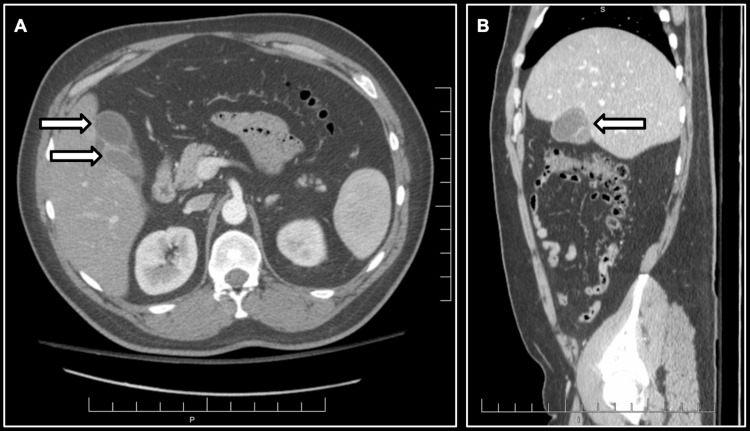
CT imaging demonstrating gallbladder duplication with acute cholecystitis (A) Axial contrast-enhanced CT image of the abdomen shows two fluid-filled, tubular structures in the right upper quadrant (white arrows), located in the gallbladder fossa. (B) Coronal reformatted CT image shows the duplicated gallbladders oriented vertically beneath the liver (white arrow), confirming their distinct morphology and alignment
